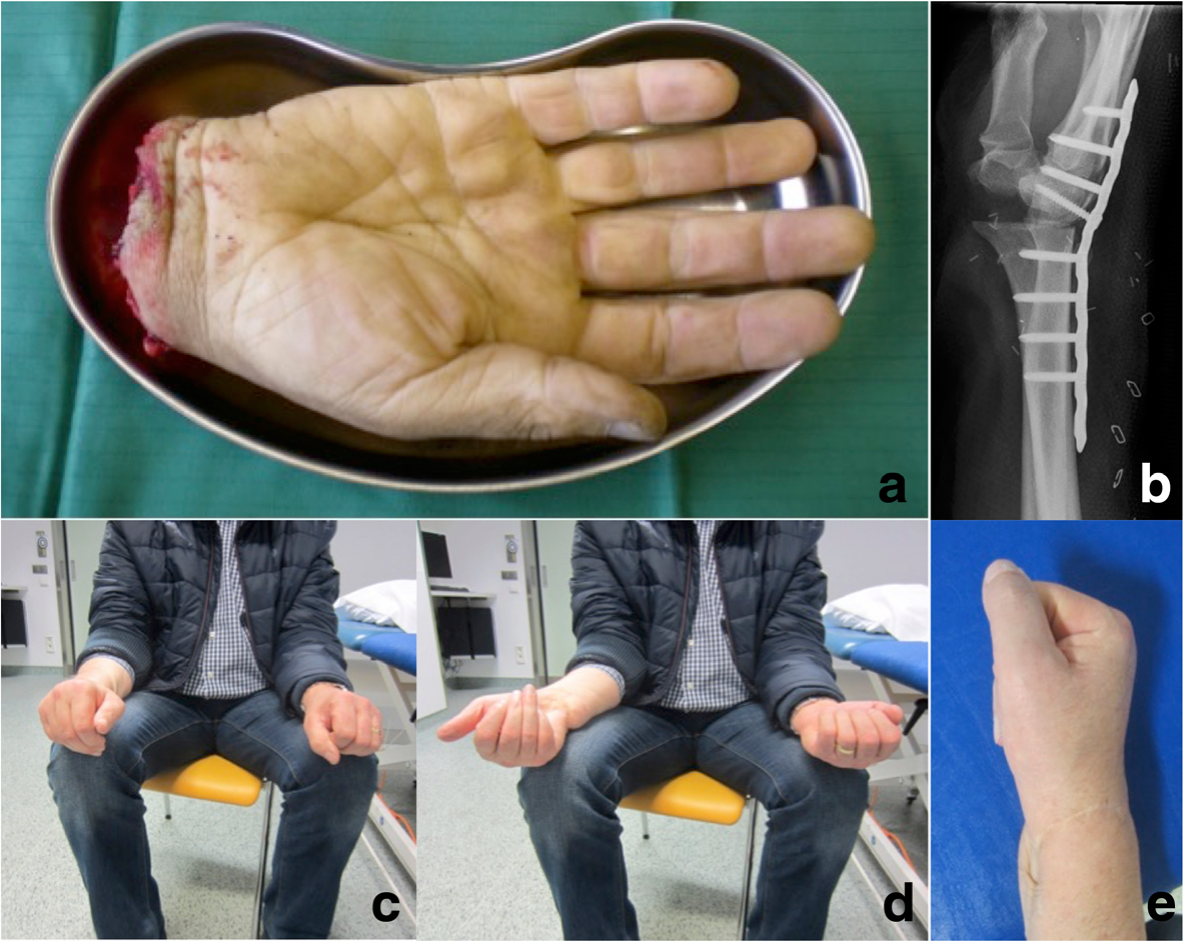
Case three – Total amputation at the level of the wrist joint. a preoperative picture of the amputate b post-operative X-ray after arthrodesis of the wrist-joint. c Slightly reduced flexion of the fingers and forearm pronation on the affected *right side*. d Full range of forearm supination. e fist closure in neutral position
